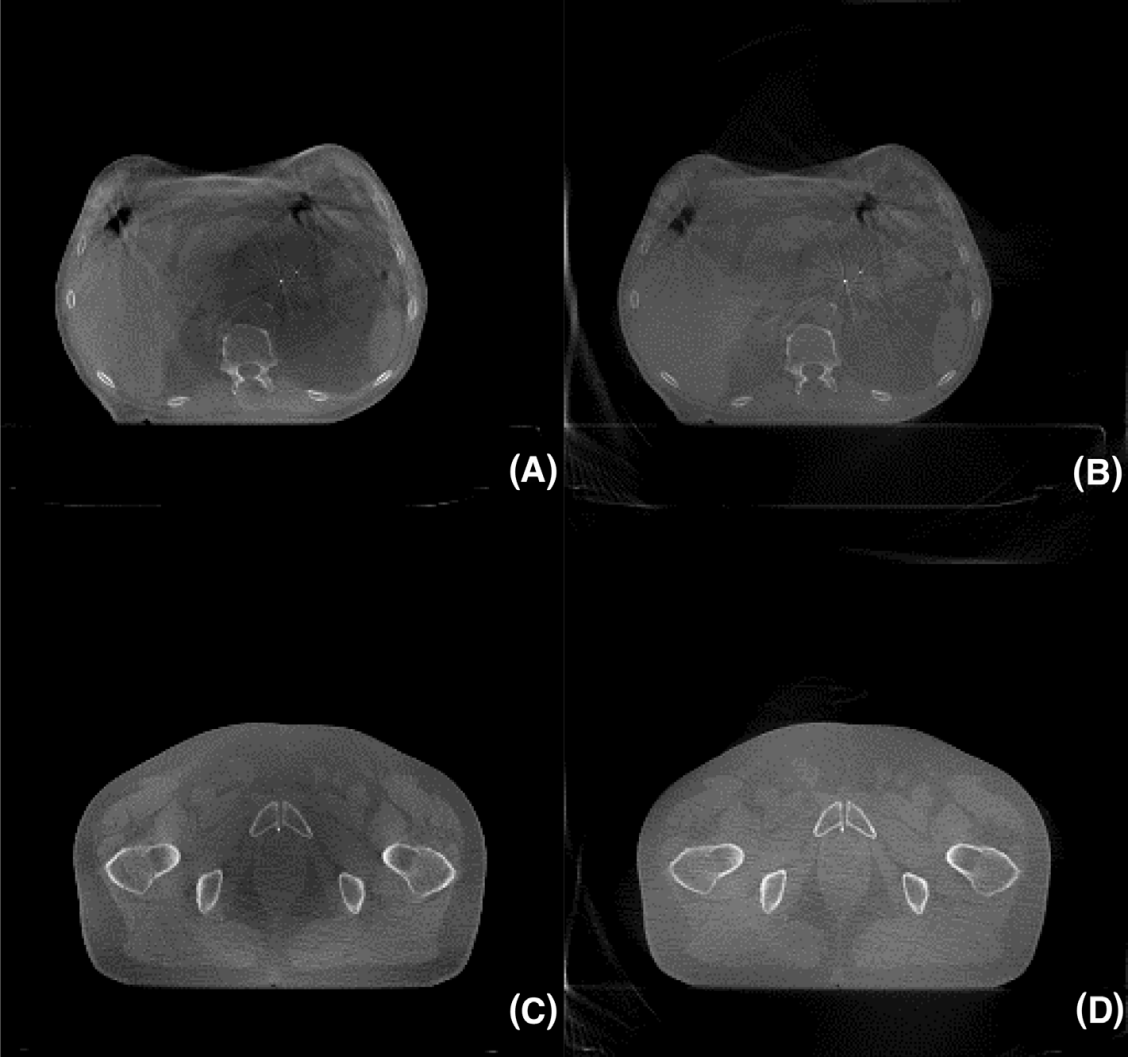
Reconstruction images of pancreatic (A), (B) and prostate (C), (D) cancer patient treated under radiation therapy. (A), (C) FDK, (B), (D) VS-SART-BB. A total of 655 x-ray projections were acquired in half-fan mode.
